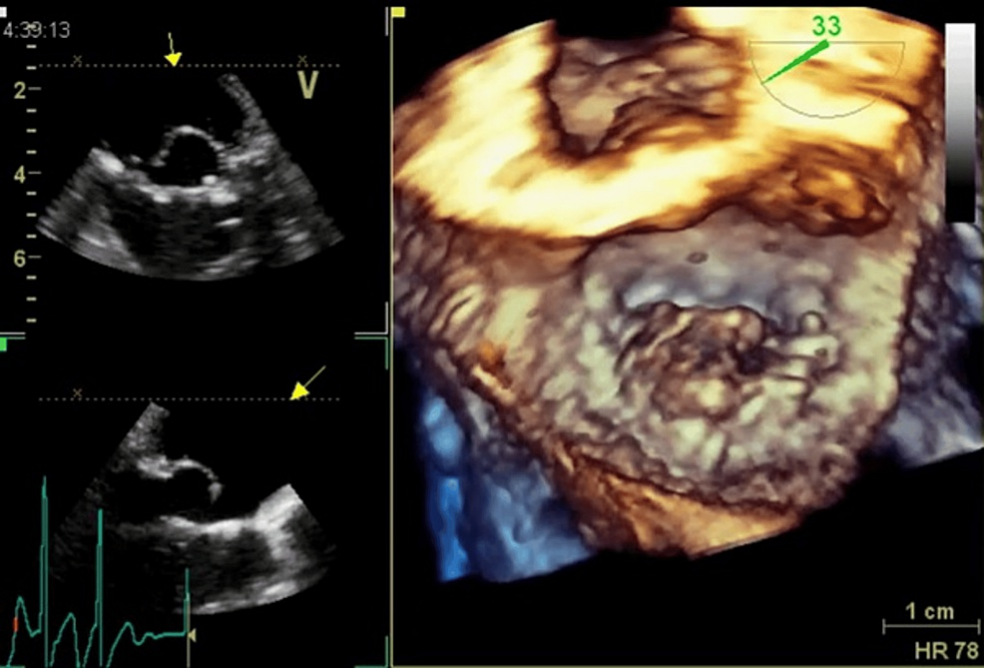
The patient’s TEE image after the termination of the first CPB Three-dimensional TEE image displays a prolapse complication in the P1 to P2 segments of the mitral valve, areas that have been reinforced with pericardial tissue. TEE, transesophageal echocardiography; P1, anterior scallop of the posterior mitral leaflet; P2, middle scallop of the posterior mitral leaflet; CPB, cardiopulmonary bypass
